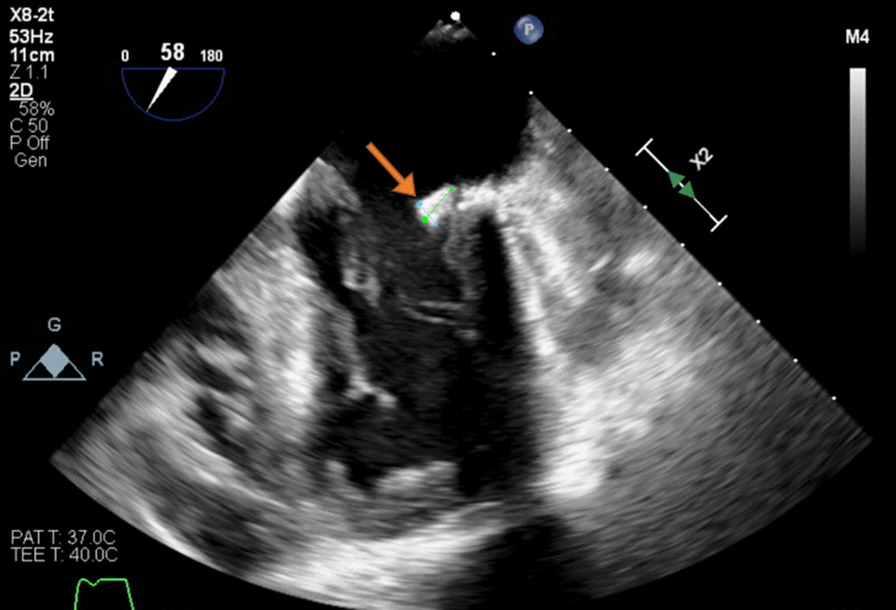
Transesophageal echocardiogram showing 0.76 cm × 0.50 cm vegetation (arrow) on the Posterior mitral valve leaflet
